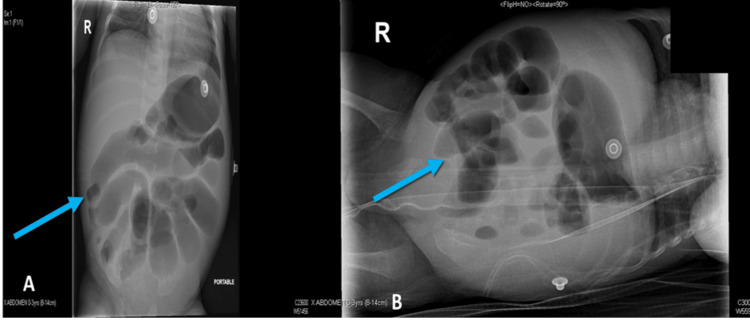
X-ray abdomen, two views: A. Anterior-posterior view showed massive thickened, dilated bowel loops (blue arrow). B. The lateral decubitus view showed no features of pneumatosis intestinalis (blue arrow)
